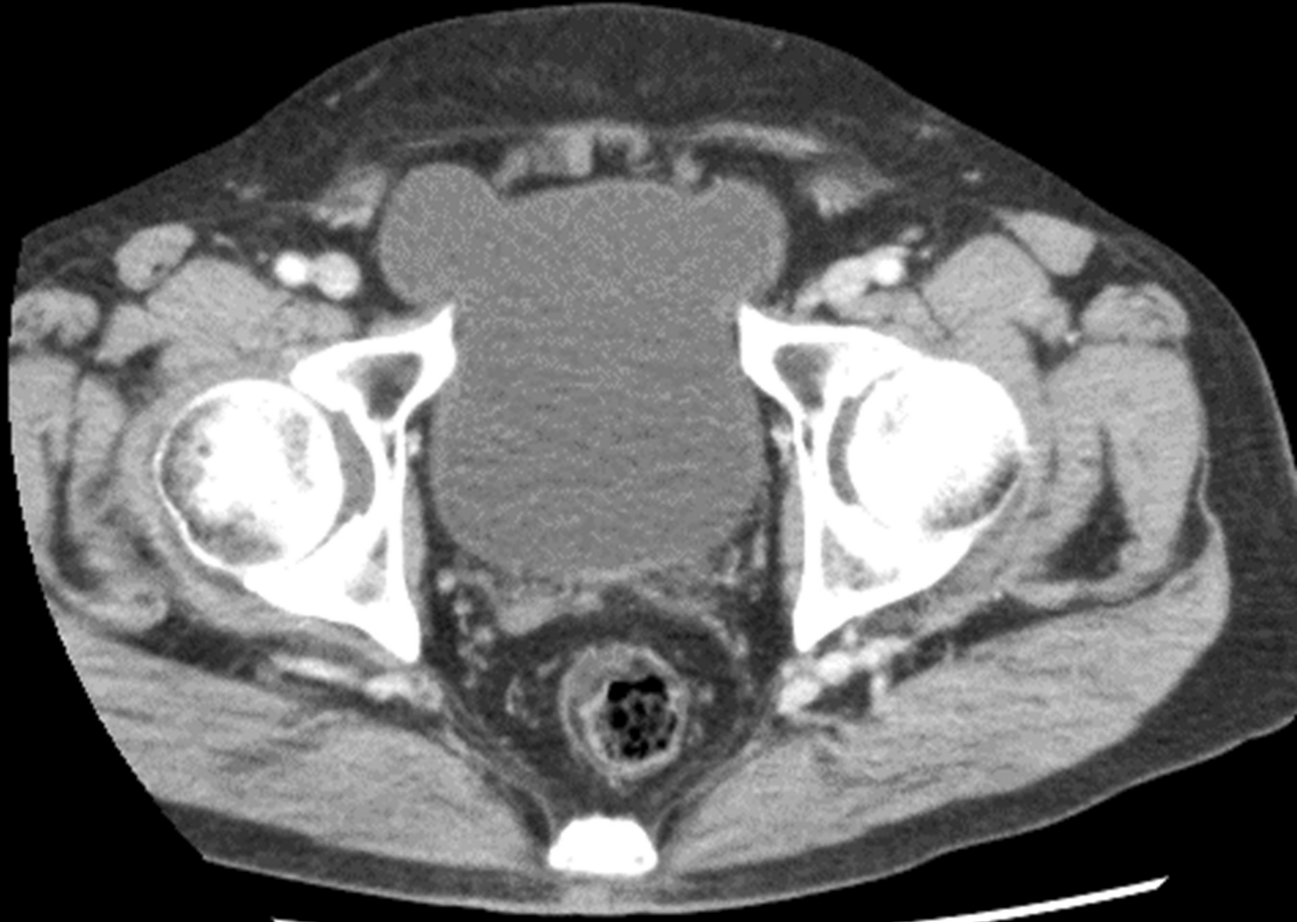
Contrast‐enhanced computed tomography of the abdomen. Bilateral bladder hernias are shown as fluid‐filled structures continuous with the bladder
